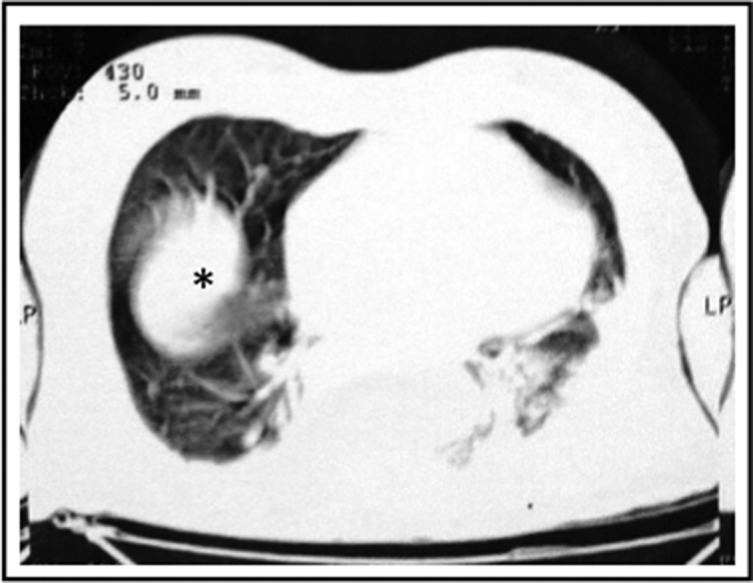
Computed tomography of the abdomen showing a mass with fluid-retaining in the central and left cavity of the lung, and thick irregular wall over the right and left lung (LP). A dense mass is shown in the center of right lung (∗).
